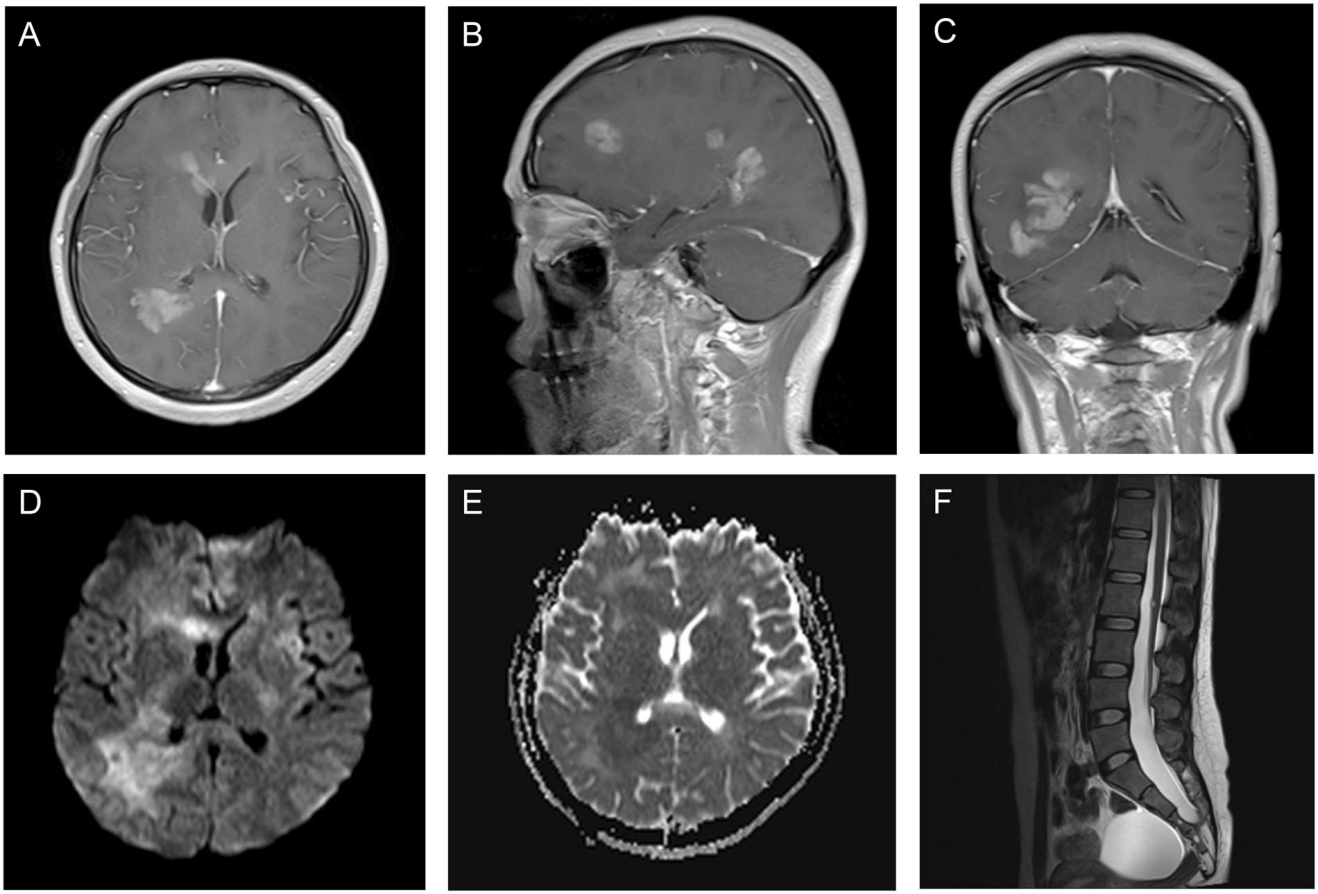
A Grade II PXA involving the right frontal lobe, right temporal-parietal lobe, corpus callosum, and left insula in a 25-year-old female. (A–C) Contrast-enhanced axial, sagittal, and coronal T1WI showing marked enhancement of multiple lesions in the brain. (D) DWI showing restricted diffusion of multiple lesions. (E) The mean ADC of the right temporal-parietal lobe lesion was 0.80 × 10^−3^ mm^2^/s, and the mean ADC ratio was 1.01. (F) Sagittal T2WI depicting a hyper-intense spinal intramedullary lesion at the L2 level.
